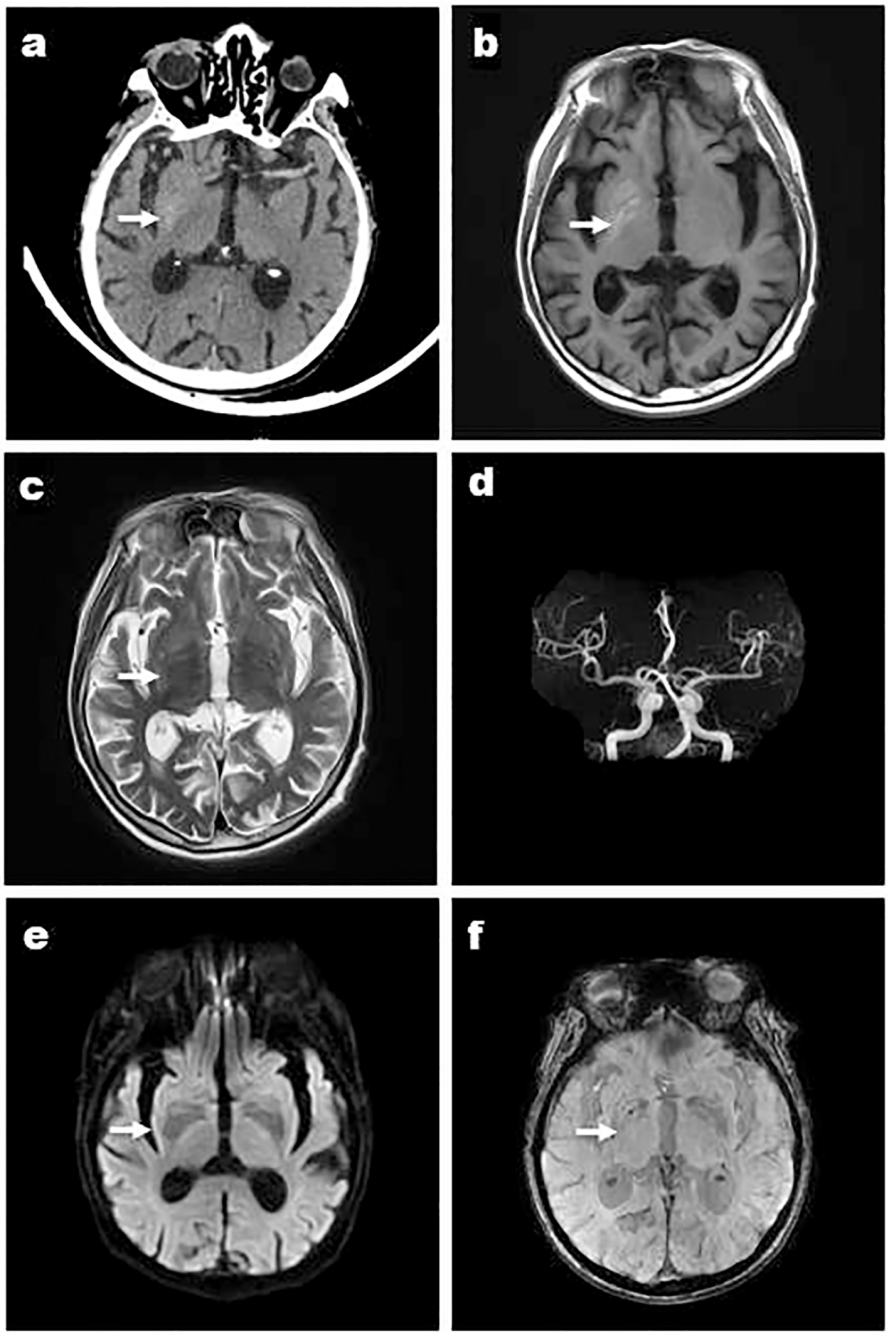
Case 6, a 84-year-old male, was admitted with “left limb involuntarily writhing for 5 days”and images showed that the right striatum of involuntary movements was high density on CT (A), high signal on T1WI (B), iso-signal on T2WI (C), and MRA showed arterial stenosis (D). DWI didn’t show high signal (E) and a low signal in the right basal ganglia was shown in susceptibility weighted imaging (SWI) (F). Arrows indicate striatum abnormal signal.
